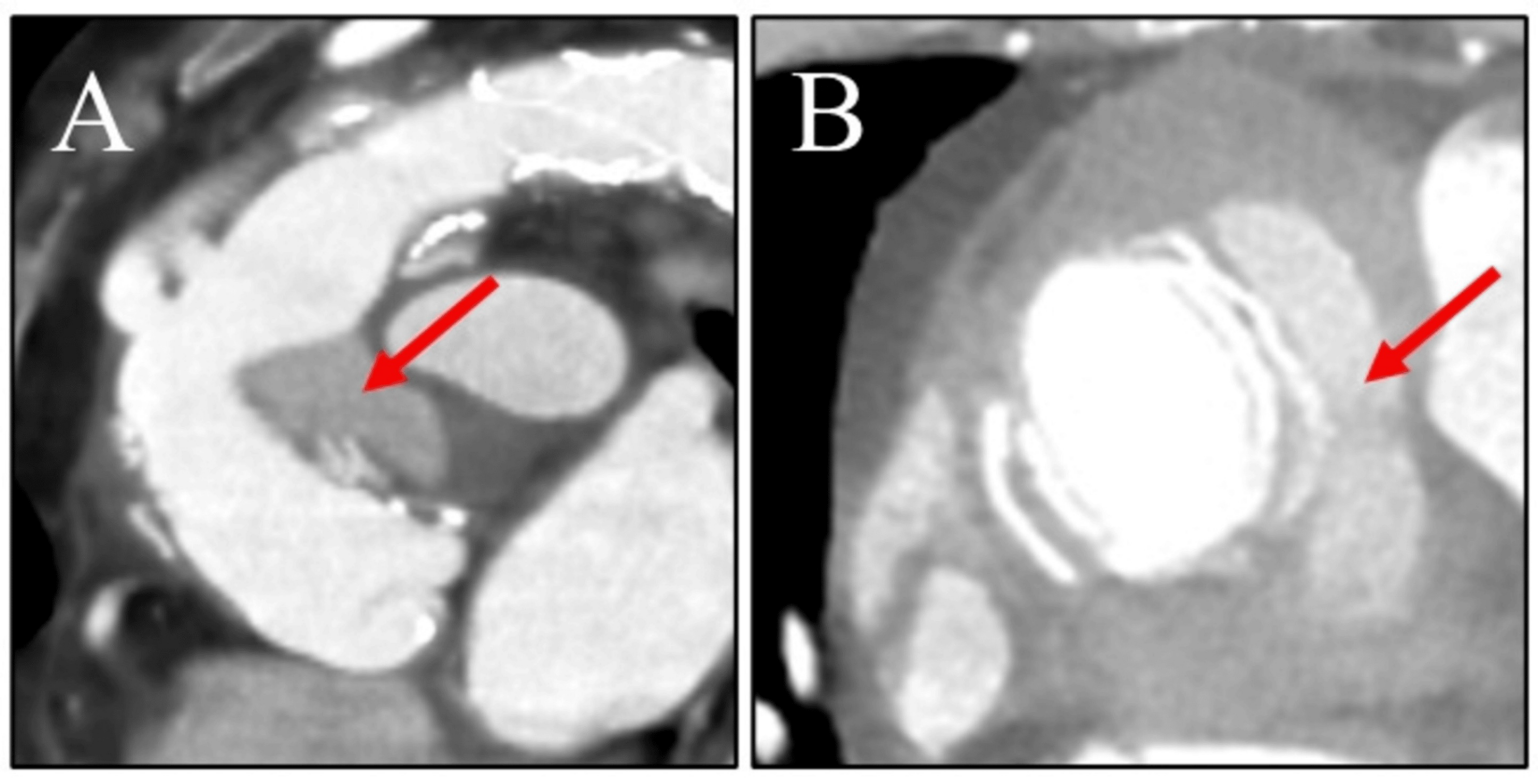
Preoperative computed tomography findings Panels A and B showed an impending rupture of the pseudoaneurysm at the graft anastomosis (arrows)
